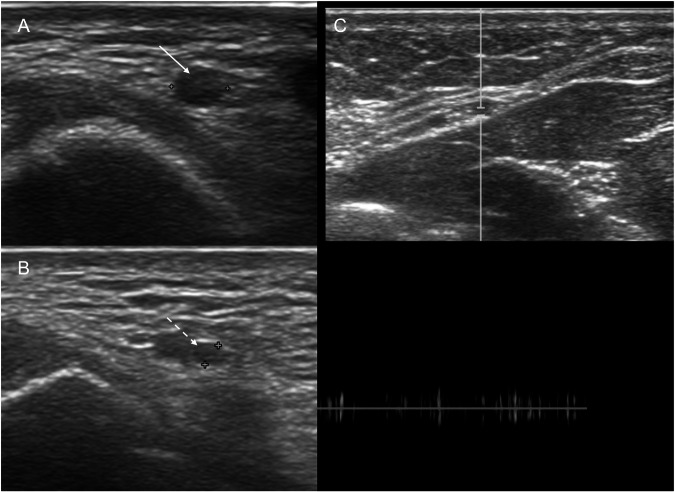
Images of a 12-year-old girl who underwent embolization of choroidal arteriovenous malformation via transradial approach. A Transverse US image obtained at the time of intervention shows patent right radial artery at the wrist level, measuring 2.2 mm. B Transverse US image obtained 1 year after intervention shows a reduction in the size of the radial artery at the wrist, measuring 1.0 mm. C Doppler US of the right forearm demonstrates a small radial artery with loss of arterial waveform and antegrade flow, confirming radial artery occlusion
